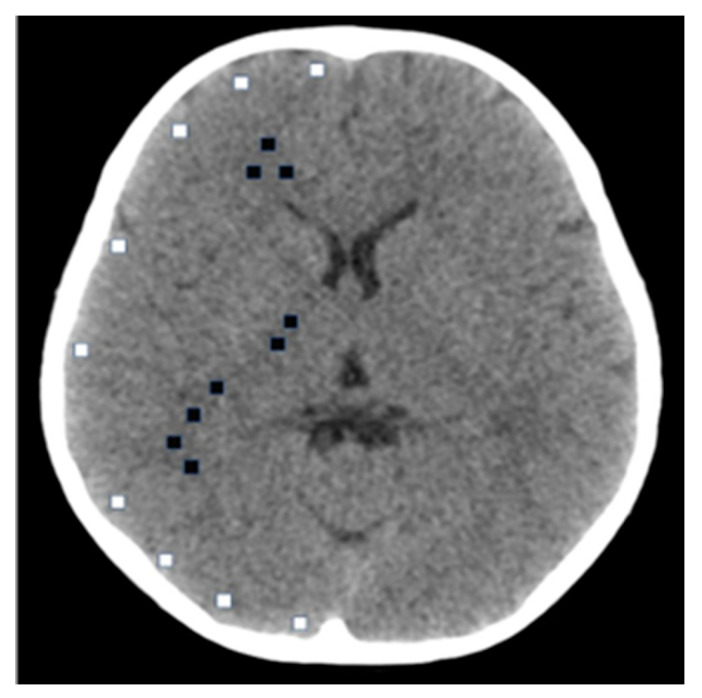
ROI placements for quantitative analysis of image quality in pediatric head CT. Multiple circular ROIs (10 × 10 pixels) were placed in the gray and white matter regions at the level of the basal ganglia. The mean CT values and standard deviations across all ROIs were used to calculate the SD and contrast-to-noise ratio (CNR) according to Equations (3) and (4).
